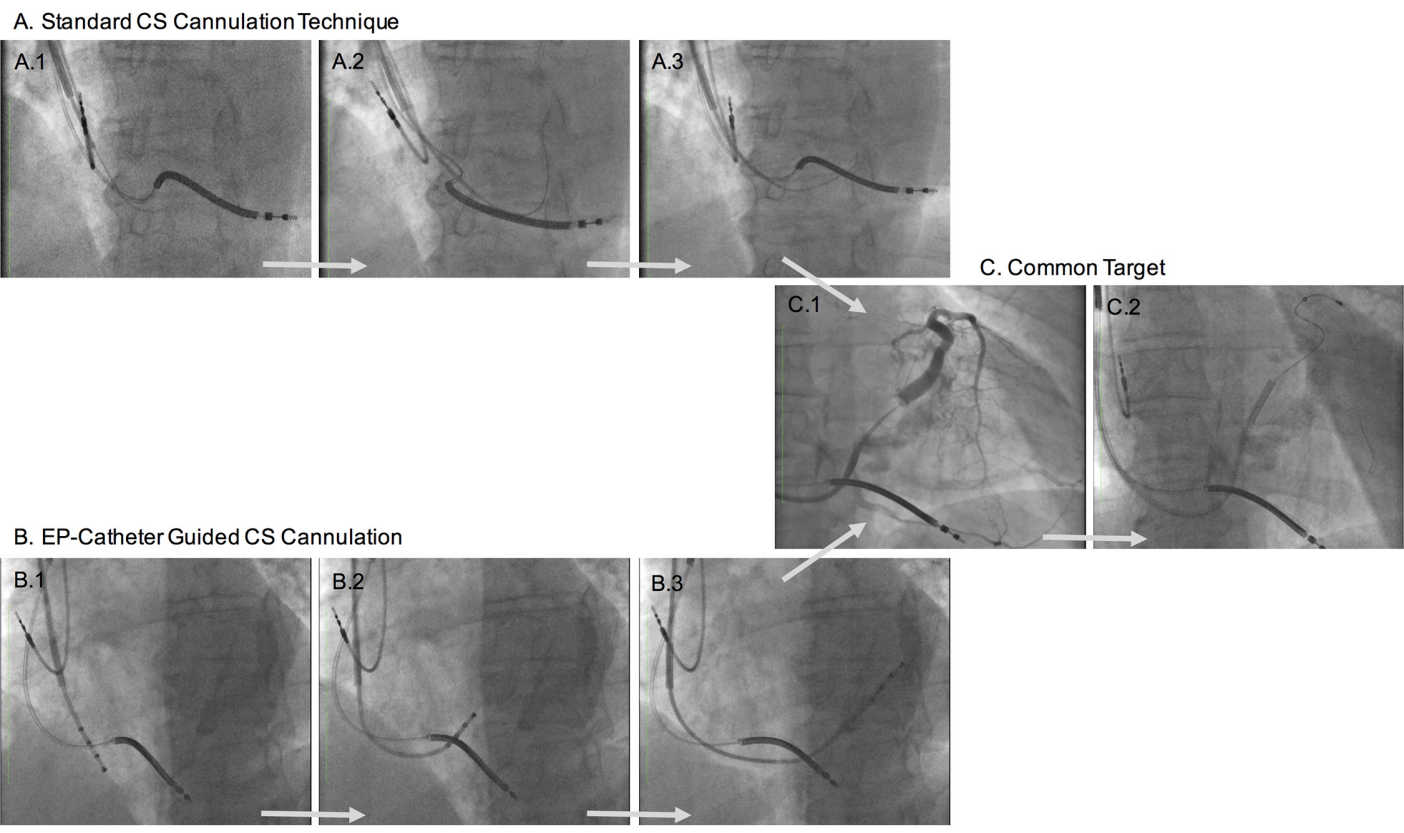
Original X-ray showing CS cannulation in LAO 30° view. 1. A.1-3. Standard cannulation technique using preformed sheets and hydrophilic wire. B.1-3. Steerable EP catheter with exact cannulation. C.1-2. CS angiogram and coronary wire guided LV-lead positioning.
